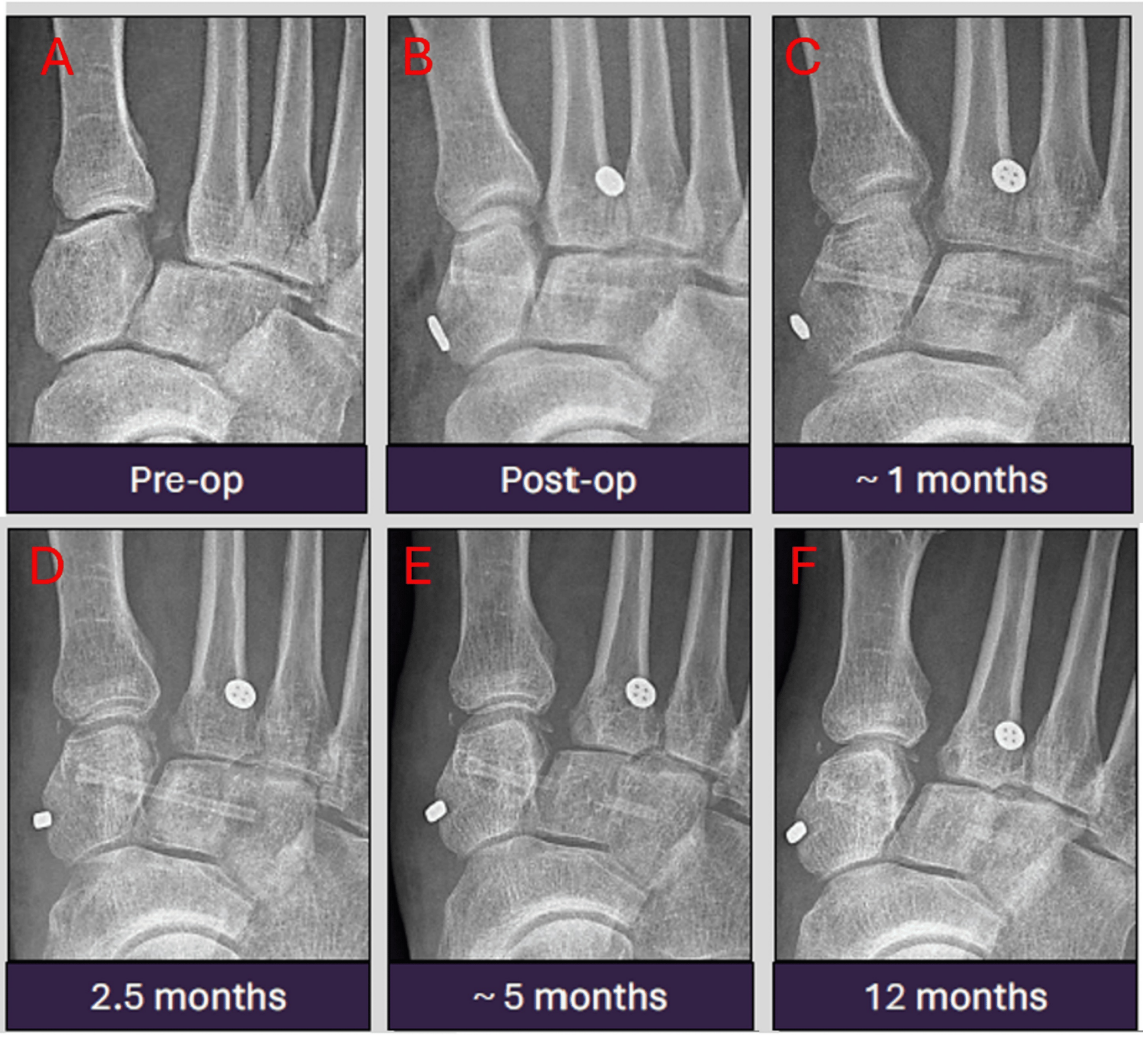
Serial antero-posterior radiographic images from pre-operation to 12 months post-operation showing expected progressive implant fragmentation and degradation without migration or loss of reduction. The radiographs were taken at pre-operation (A), immediately post-operation (B), one month (C), 2.5 months (D), five months (E), and 12 months (F) post-operation.
